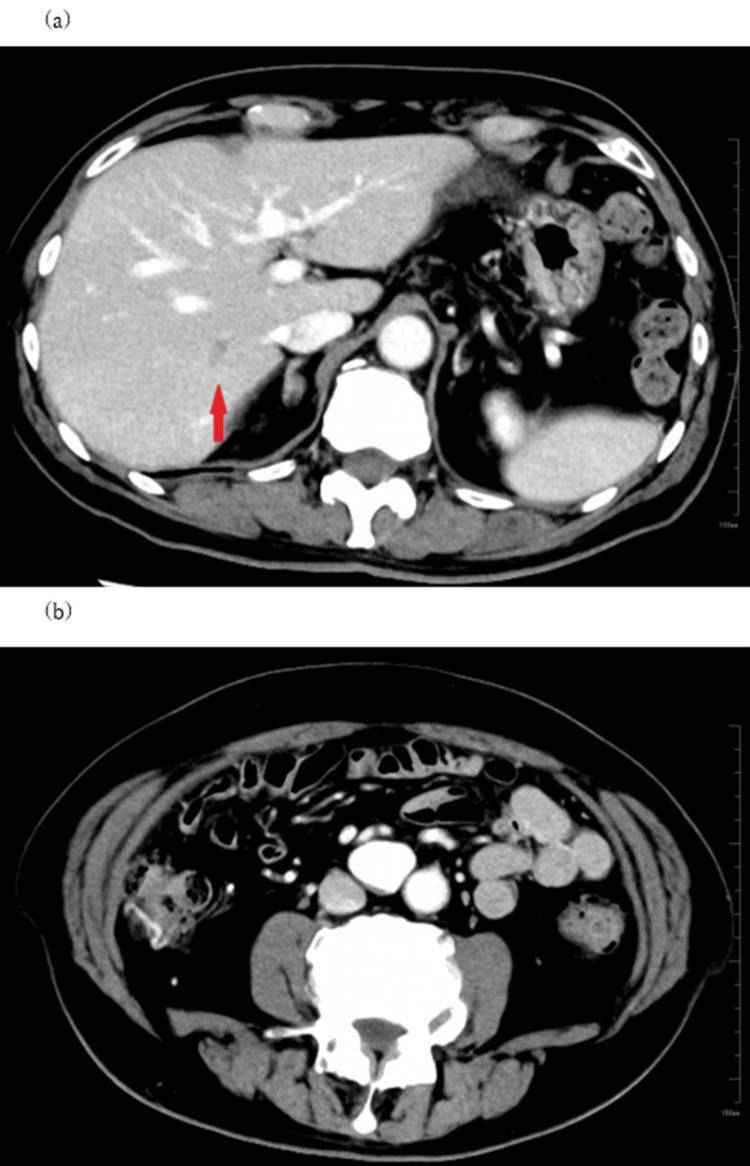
Axial view of the enhanced abdominal CT after one year. Intraluminal thrombus in the superior mesenteric vein (SMV) aneurysm has disappeared at this point (a), while an embolus of the intrahepatic portal vein (arrow) is revealed (b).
